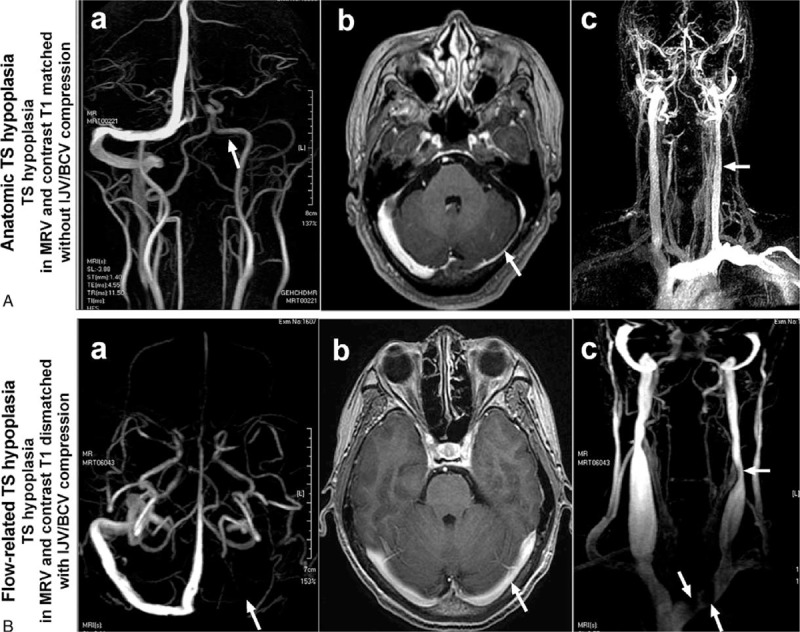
The concordance and discrepancy of transverse sinus (TS) in MRV and contrast T1-weighted magnetic resonance imaging (contrast T1). (A) Left TS in MRV (a, arrow) and contrast T1 (b, arrow) match in a study subject without IJV/BCV compression (c, arrow). (B) Left TS in MRV (a, arrow) and contrast T1 (b, arrow) mismatch in a study subject with IJV/BCV compression (c, arrows).
